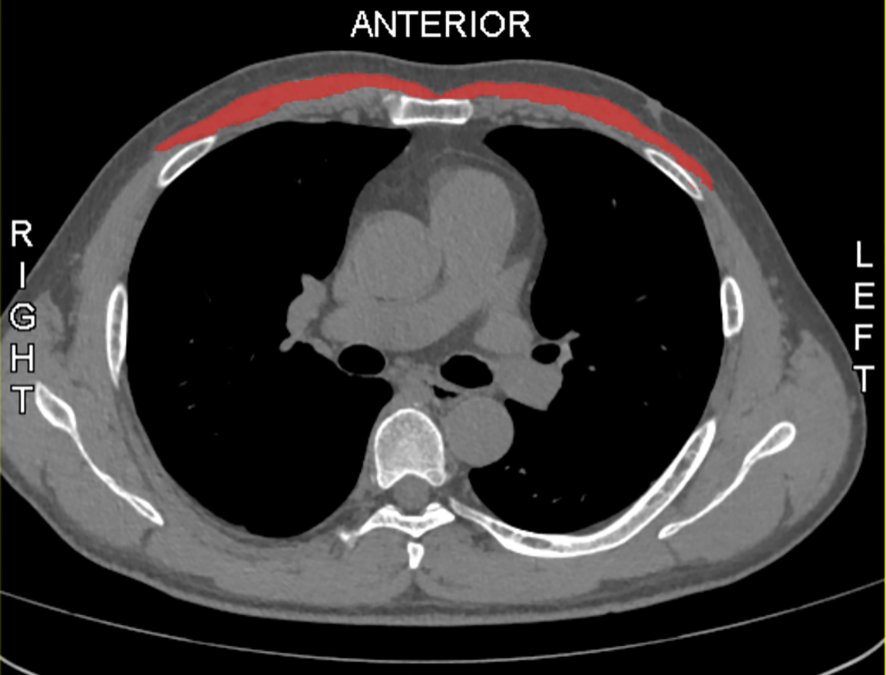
Sample axial CT images of the sixth thoracic vertebral region. Pectoralis muscles are in red.
